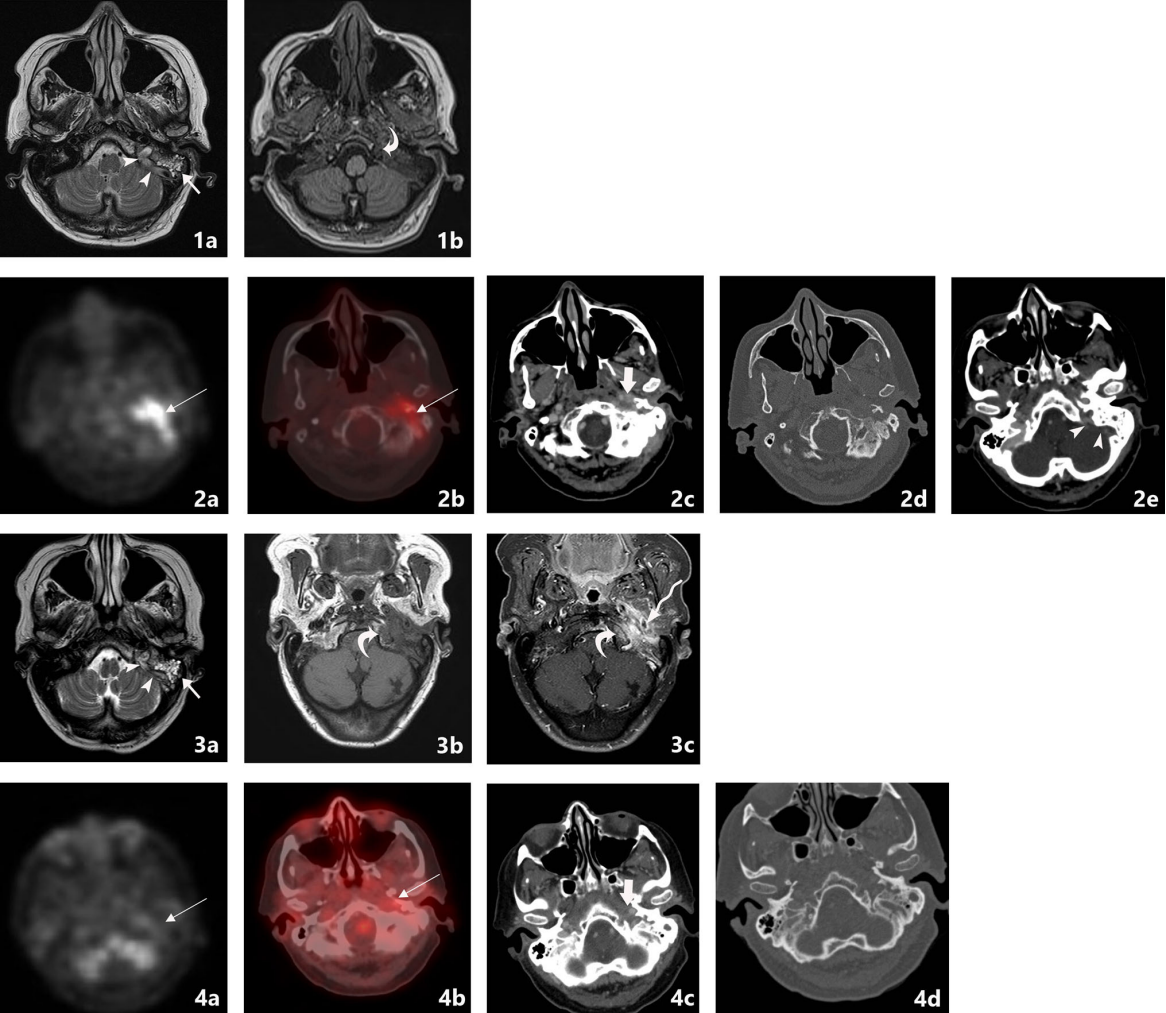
Transverse sinus thrombosis. A 76-year-old female presented with pain and otorrhea of the left ear since 2 months, with hearing loss and tinnitus. The symptoms were accompanied by a left-sided headache. No cranial nerve deficit was present. No history of diabetes or immune suppressive disease was present. Culture from the EAC revealed *Pseudomonas* as causal agent. An MRI was made at presentation with T2-w (image 1a) and T1-w (image 1b) series. At T2-w (image 1a) high signal intensity is present in the mastoid air cells at the left side (straight arrow), with loss of flow voids of the sigmoid sinus (arrowheads) indicative for sinus thrombosis. T1-w images show loss of signal intensity of the bone marrow of the skull base (image 1b, bent arrow), consistent with SBO. FDG-PET-CT performed 3 weeks after initial diagnosis confirms the location of SBO with increased uptake at the left temporal bone and surrounding soft tissue (image 2a, 2b fused PET-CT image, thin arrow). On the diagnostic CT, the decrease of subtemporal fatplanes with enhancing soft tissue at the stylomastoid foramen (image 2c thick arrow), bone erosion (image 2d) and sinus thrombosis (image 2e arrowheads) are reaffirmed. Additional MRI sequences (image 3a:T2-w; 3b:T1-w, 3c: T1-w fs) were executed showing added value of T1-w fatsat (fs) post-gadolineum scan (image 3c); the encasement of the internal carotid artery (arrow) is more easily appreciated within the area of extensive bone involvement (bent arrow). Follo-up FDG-PET-CT at 4 months (image 4a: PET; 4b: colour fused PET-CT; 4c: CE-CT soft tissue window; 4d: CE-CT-CT bone window) shows normalization of FDG avidity (image 4a thin arrow), normalization of enhancement of soft tissues (image 4b thin arrow) and sclerotic healing of the affected osseous tissue (image 4c thick arrow) (Color figure online)
